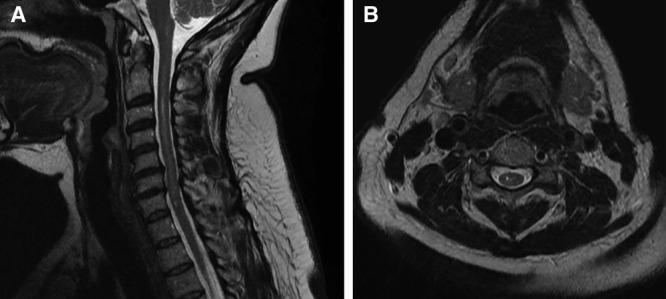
Cervical spine magnetic resonance imaging T2-weighted sagittal (A) and axial (B) images demonstrating degenerative changes and myelomalacia extending to the right dorsal medulla and cervical cord.
